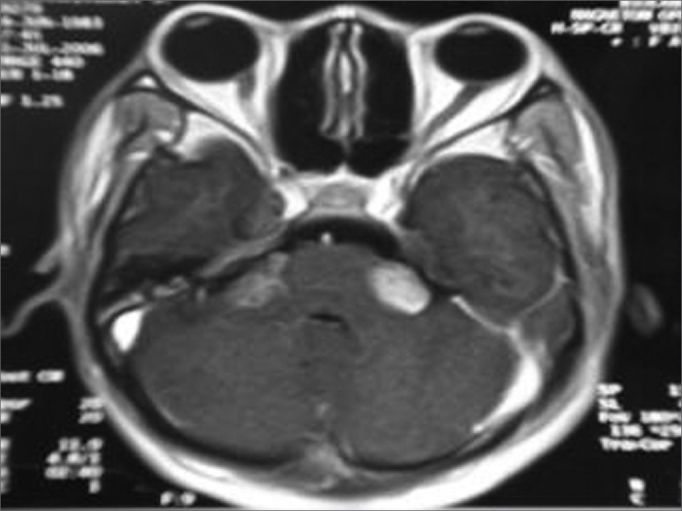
MRI (T1 contrast-enhanced) case 4 preoperative axial view.
